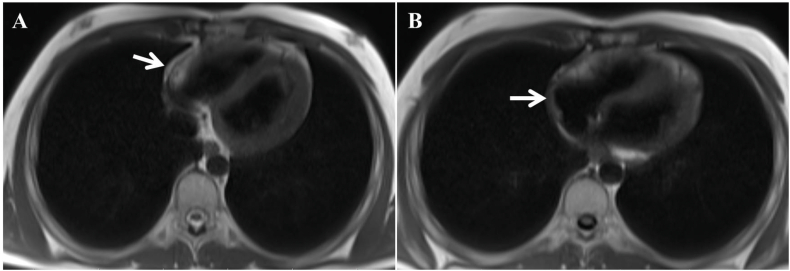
Magnetic resonance imaging at axial sections revealed (A) a stable nodular lesion (arrow), the disappearance of another nodular lesion, and (B) the absence of a new lesion at the tumor bed (arrow).
